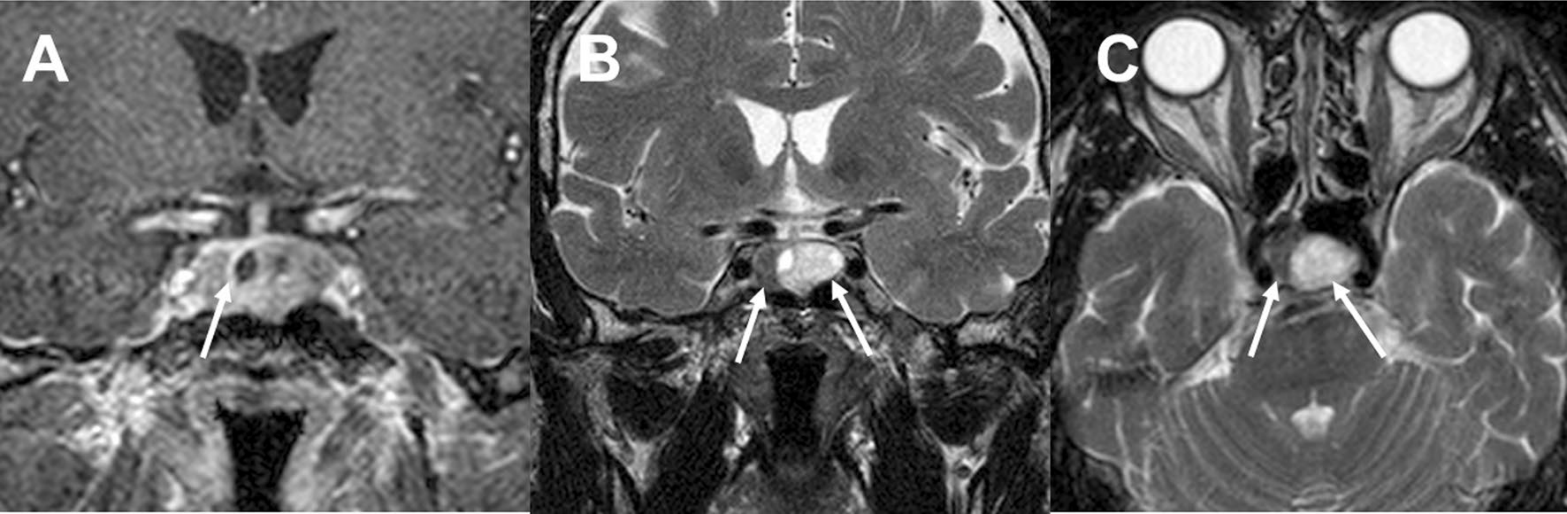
Case no. 2: a coronal T1 weighted MRI after Gd-DTPA demonstrates two clearly separate pituitary adenomas with flattened normal pituitary gland (arrow), b and c coronal and axial T2 MRI show gonadotroph macroadenoma with cystic degeneration (on the left side) and solid somatotroph adenoma (on the right side)
